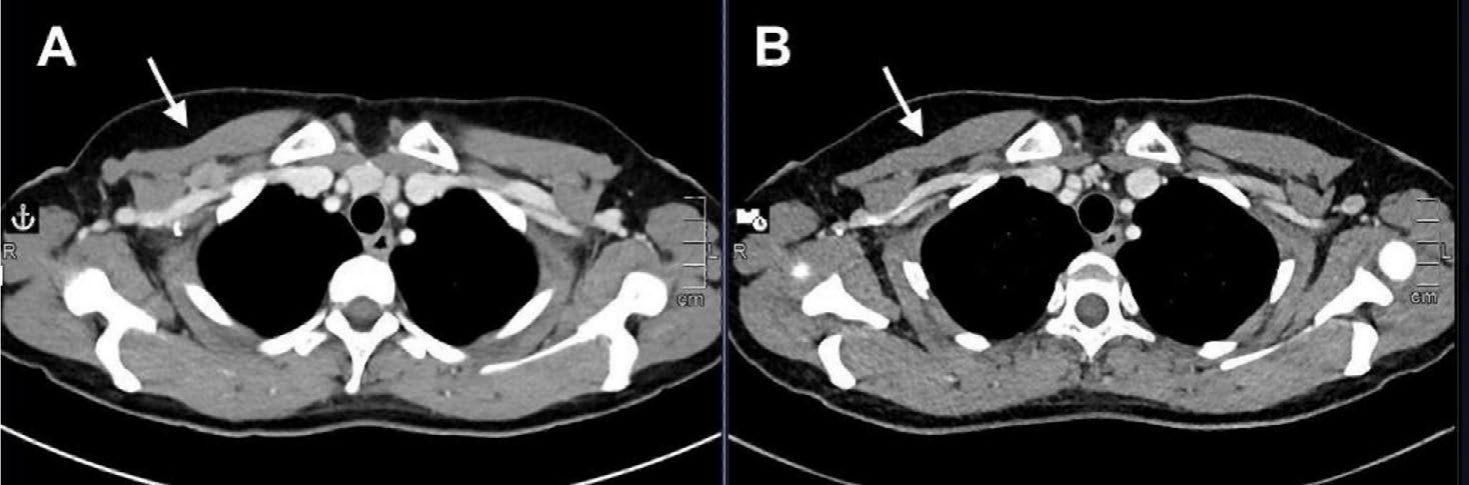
(A) Axial reconstruction of computed tomography scan showing a lymph node conglobate with a size of 20 × 12 mm (June 2019). (B) Corresponding level axial reconstruction showing the complete response.
